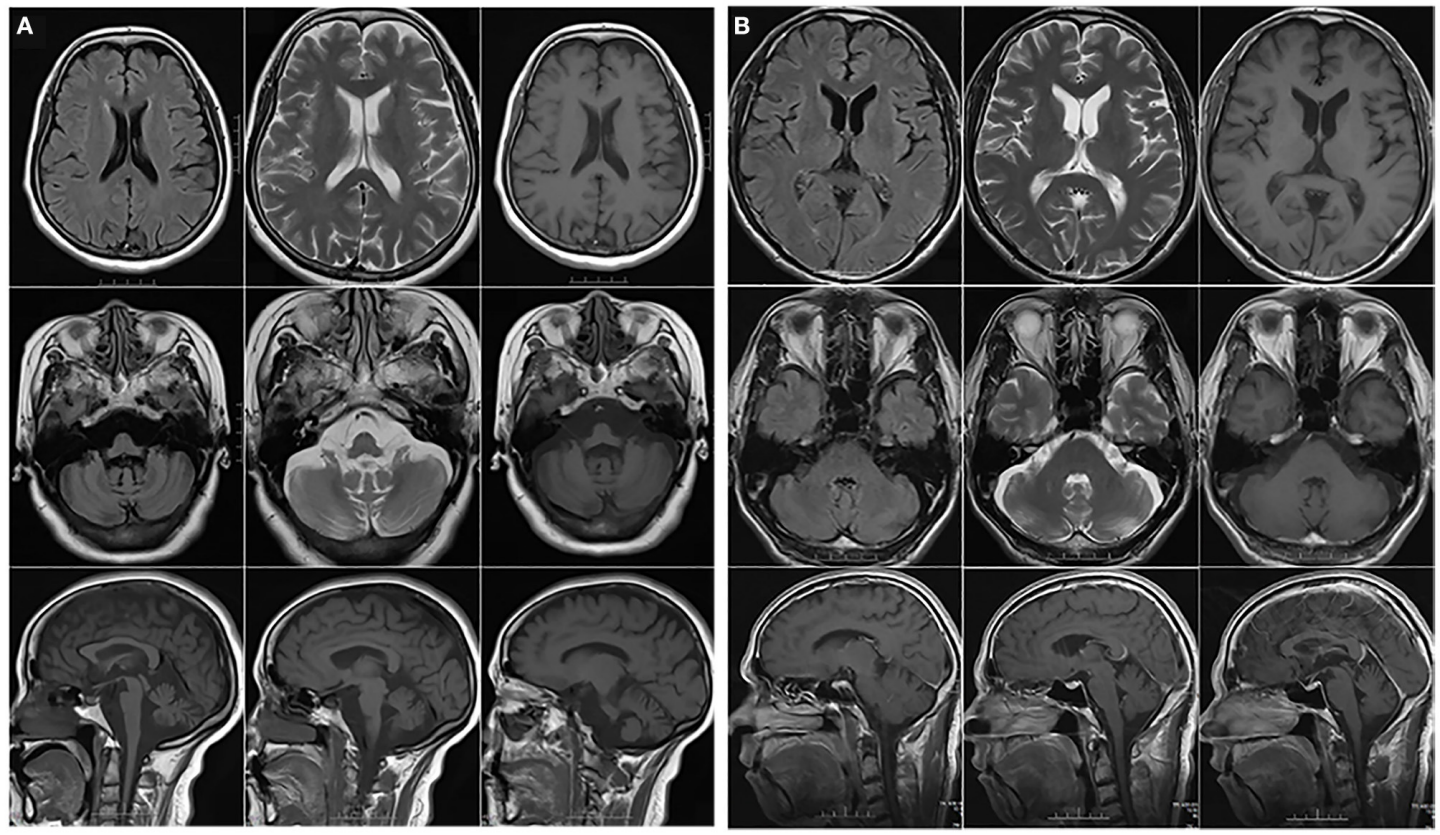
Brain magnetic resonance imaging from patient A0154 (A) and patient A002447 (B).
